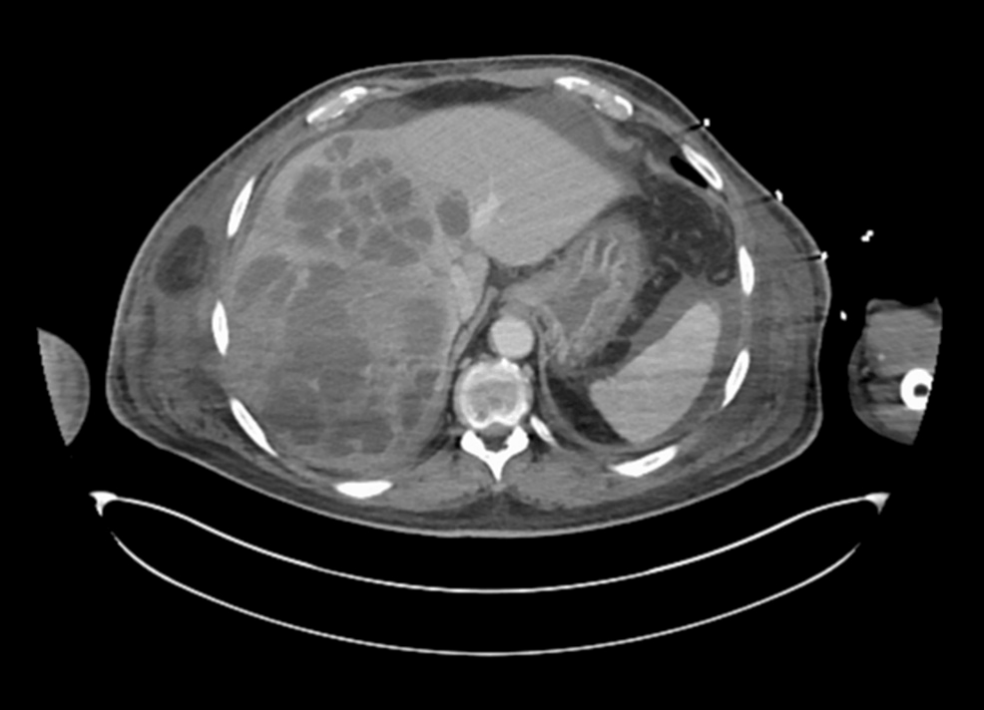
Initial CT scan of the abdomen and pelvis with IV contrast showing multiloculated cystic mass involving the majority of the right lobe of the liver. CT, computed tomography; IV, intravenous.
